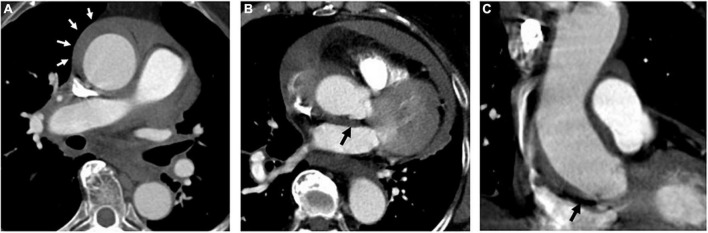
A 74-year-old woman with TAIMH and unnoticeable ULP. A contrast CT scan showed an ascending aorta aneurysm (52 mm) with a circular aortic wall thickening (9 mm) (white arrow) (A). Contrast-enhanced CT scan in the axial (B) and coronal view (C) showed an ULP (black arrow) at the ascending aorta just above the aortic root. The patient suffered from sudden death on the first day of admission. CT, computerized tomography; TAIMH, type A aortic intramural hematoma; ULP, ulcer-like projection.
